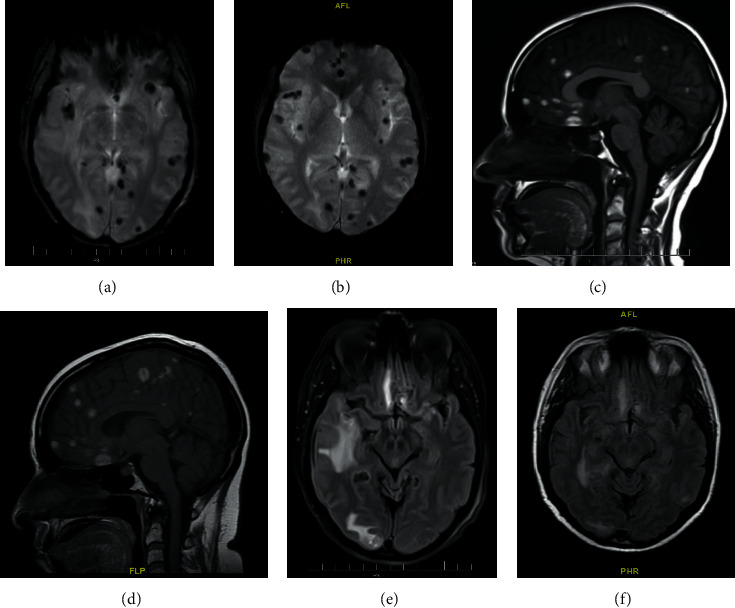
MRI brain. Innumerable foci of parenchymal hemorrhage throughout the bilateral cerebral hemispheres as well as left frontal subarachnoid hemorrhage, with mild edema and minimal local mass effect at these sites. Images (a), (c), and (e) are from a previous MRI brain (12/2020) and images (b), (d), and (f) are from the current MRI (02/2021).
